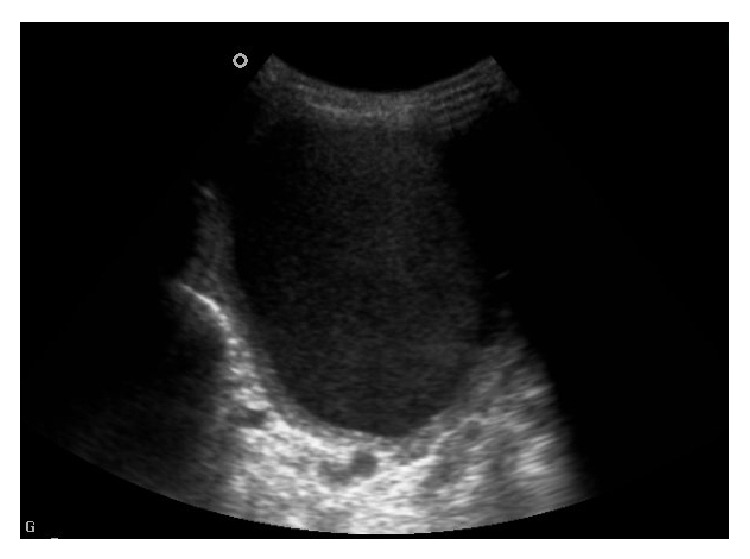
It shows cystic lesion of 10 × 8 cm in lateral left lobe of thyroid gland including internal echoes with some separation and posterior acoustic shadowing in ultrasound examination.
